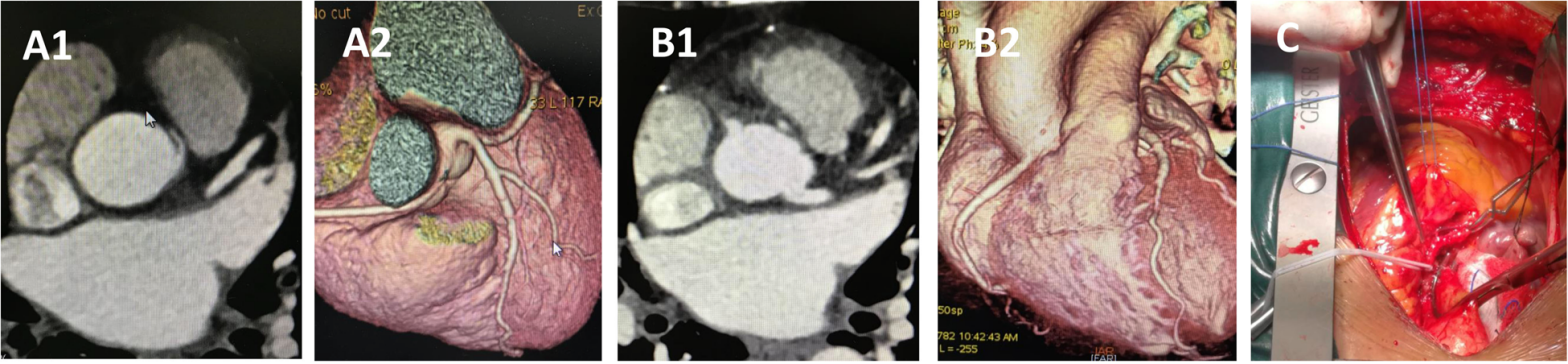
CCTA of case 10 underwent reimplantation. (A) Preoperative images showing anomalous right coronary artery arising from the left sinus. (B) Follow-up images showing proximal right main coronary artery without visible stenosis. (C) Intraoperative picture showing the reimplantation procedure without cardiopulmonary bypass. CCTA, coronary computed tomography angiography.
